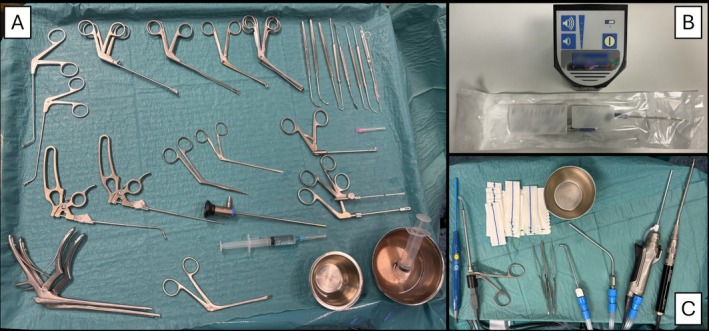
Surgical instrumentations, with cold instruments (A), doppler probe and unit (B), and powered and suction devices (C).
